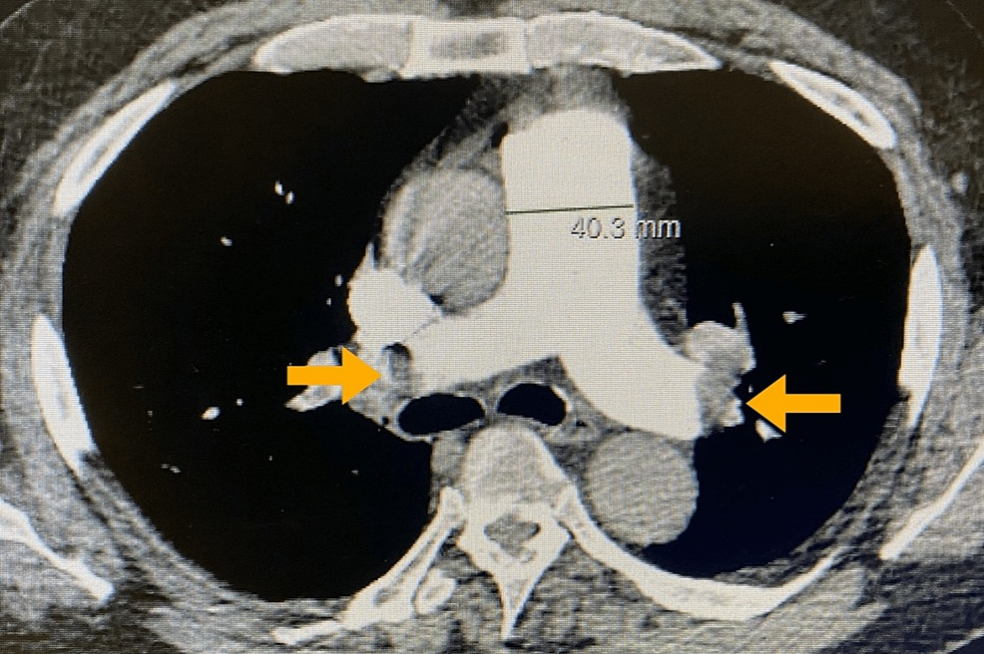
Bilateral pulmonary embolism CTA chest with contrast demonstrates filing defects in bilateral pulmonary arteries indicated by the arrows, suggesting pulmonary embolism. Pulmonary artery diameter is greater than 4 cm, suggesting pulmonary artery aneurysm.
